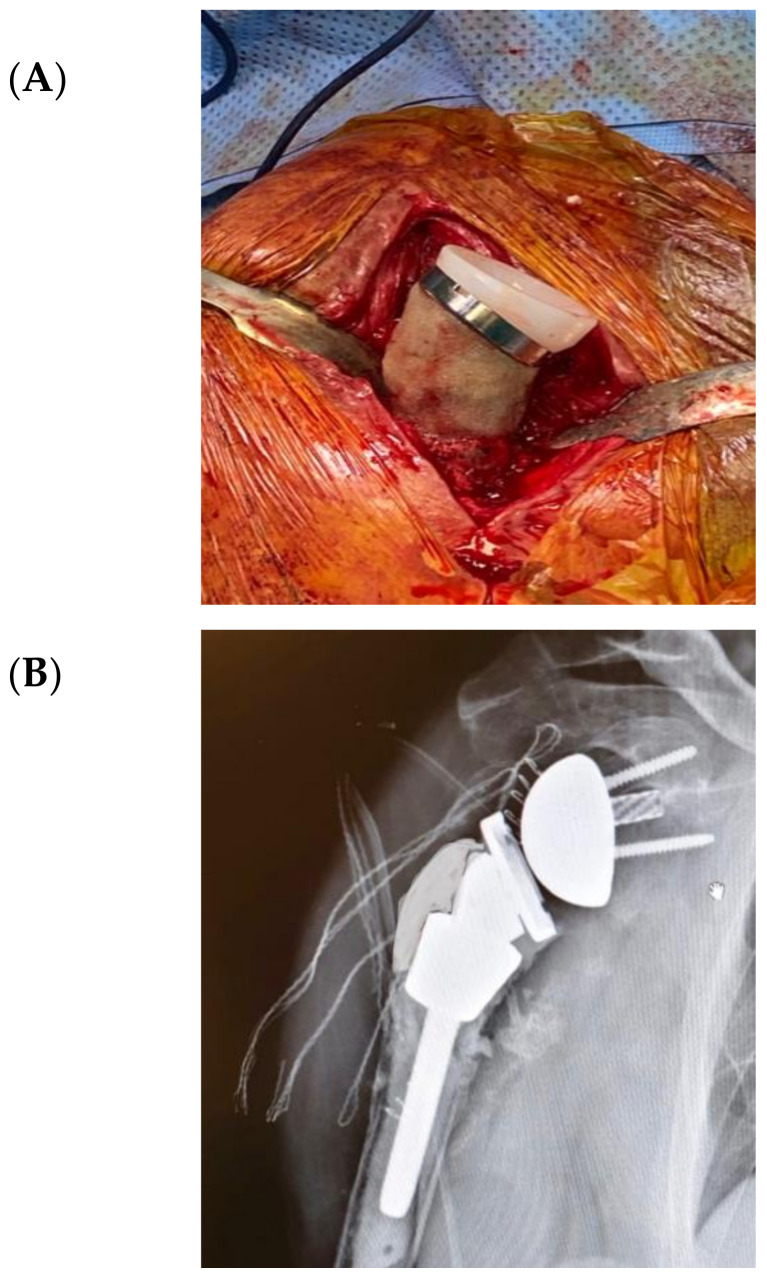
Revision final result. (A) Introperative image; (B) Postoperative X-Ray.
